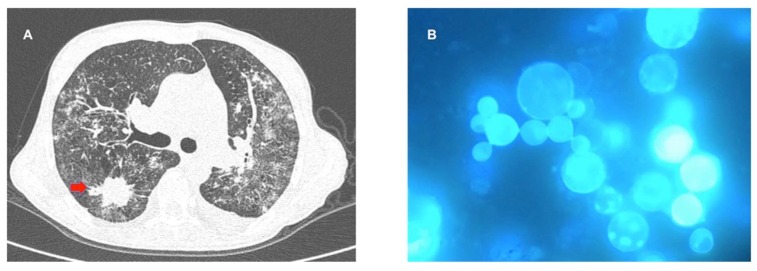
(A) Chest computed tomography scan showing spiculated nodule from adenocarcinoma (red arrow) and bilateral diffuse bronchoalveolar infiltrates from PCM. (B) Bronchoalveolar lavage with yeast cells with multiple daughter buds (Calcofluor white, 1000×). Figure A provided by Dr. Drielle Peixoto Bittencourt from Hospital do Cancer, Universidade de São Paulo; Figure B provided by the authors.
